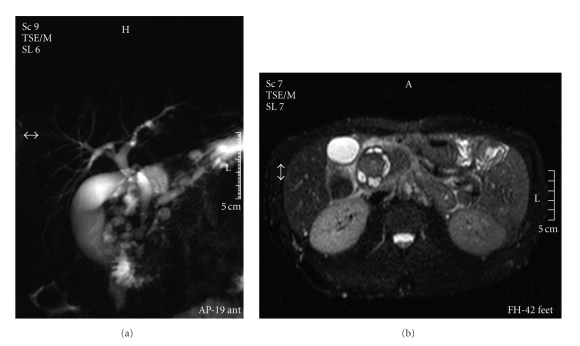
MRCP and MR images showing thickening of the second part of the duodenum, cystic formation on the duodenal wall, longitudinal narrowing of the pancreatic duct, and a mild dilatation of the common bile duct.
